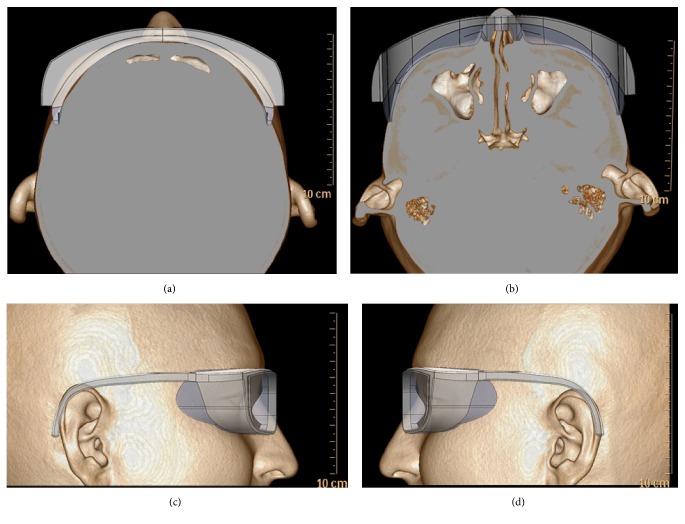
Personalization of moisture chamber spectacles using CT scan images. (a) Top, (b) bottom, (c) right side, and (d) left side.
